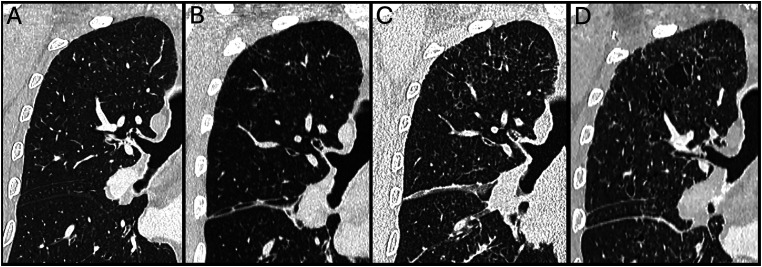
Coronal chest CT images with lung windowing, focused on the upper left lobe, show rapid progression of cystic lesions. (a) Baseline scan; (b) 2 months later; (c) 2 months and 10 days and (d) 3 months demonstrating a progressive increase in the number and size of thin-walled cysts.
